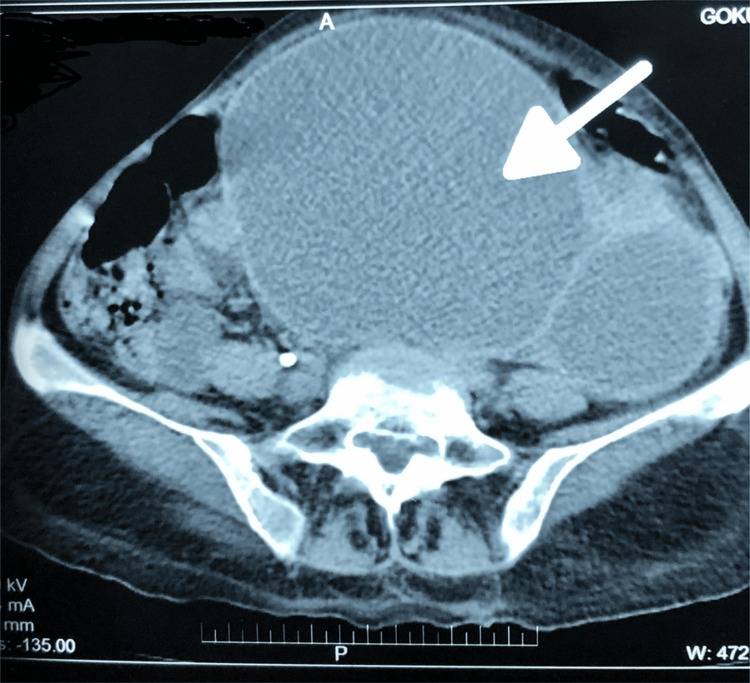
CT scan of the abdomen revealing an ectopic, grossly hydronephrotic left kidney crossing the midline (white arrow).
